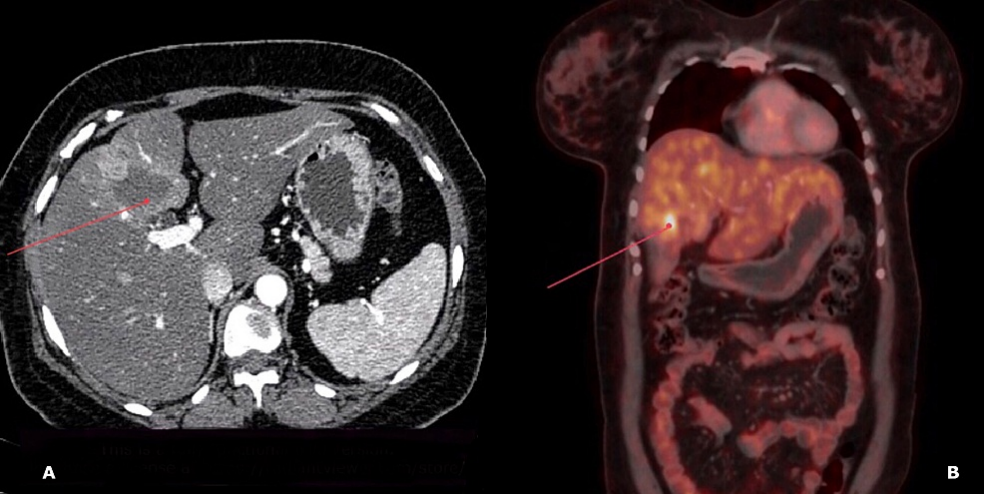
CECT(A) and PET-CT(B) images showing shrinkage of the size of tumor post neoadjuvant chemotherapy (red arrows)
